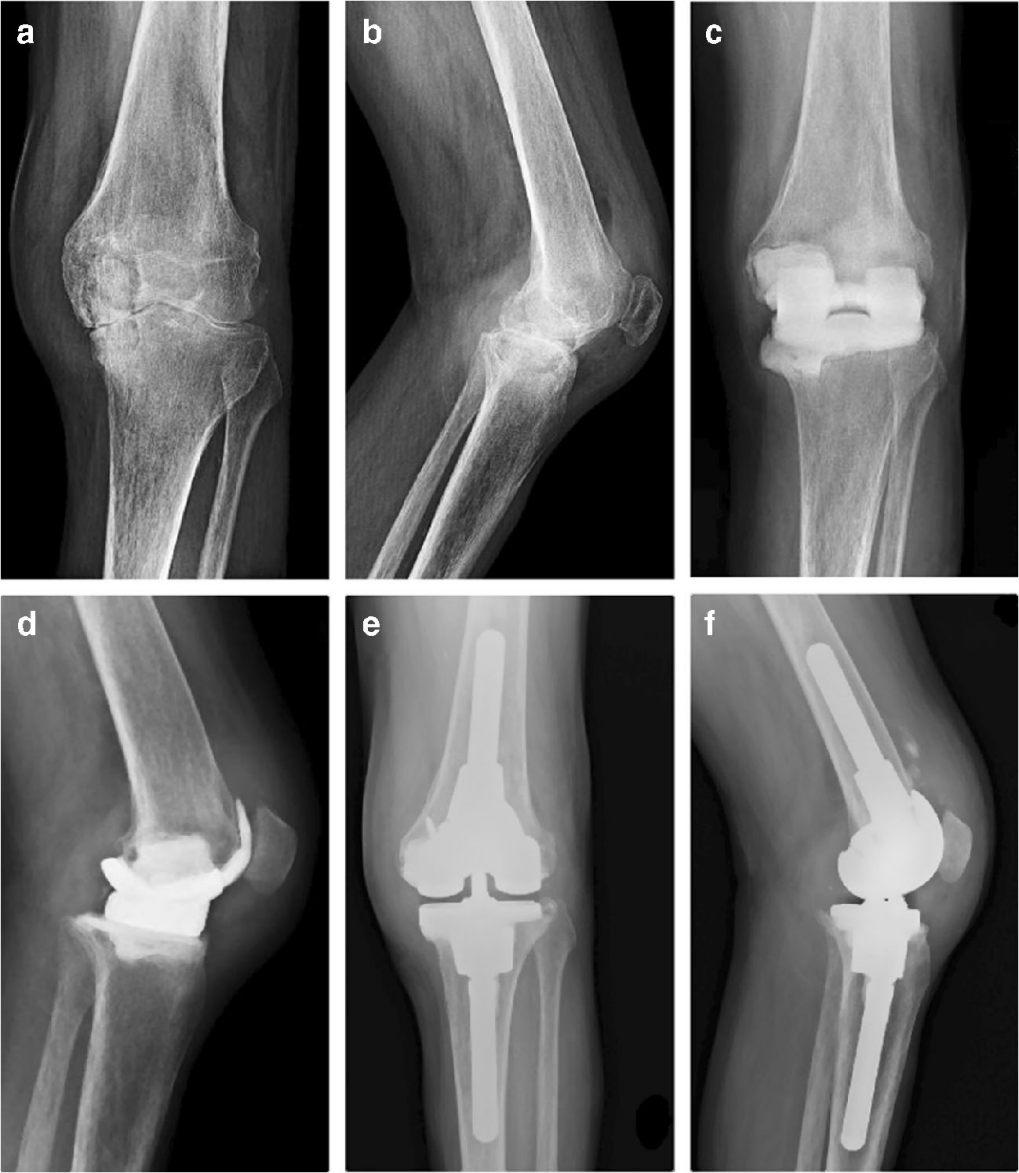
Images of a 64-year-old male patient suffering from a tubercular SA of the left knee. a, b AP and lateral view of the left knee demonstrating the wide degenerative process and deformity resulting from the infection. c, d AP and lateral view of the knee 3 months after the prefabricated articulating cement spacer was positioned showing a wide cavitary bone defect on the medial femoral condyle. e, f AP and lateral radiographs at final follow-up. The medial femoral condyle was reconstructed using a tantalum acetabular augment
